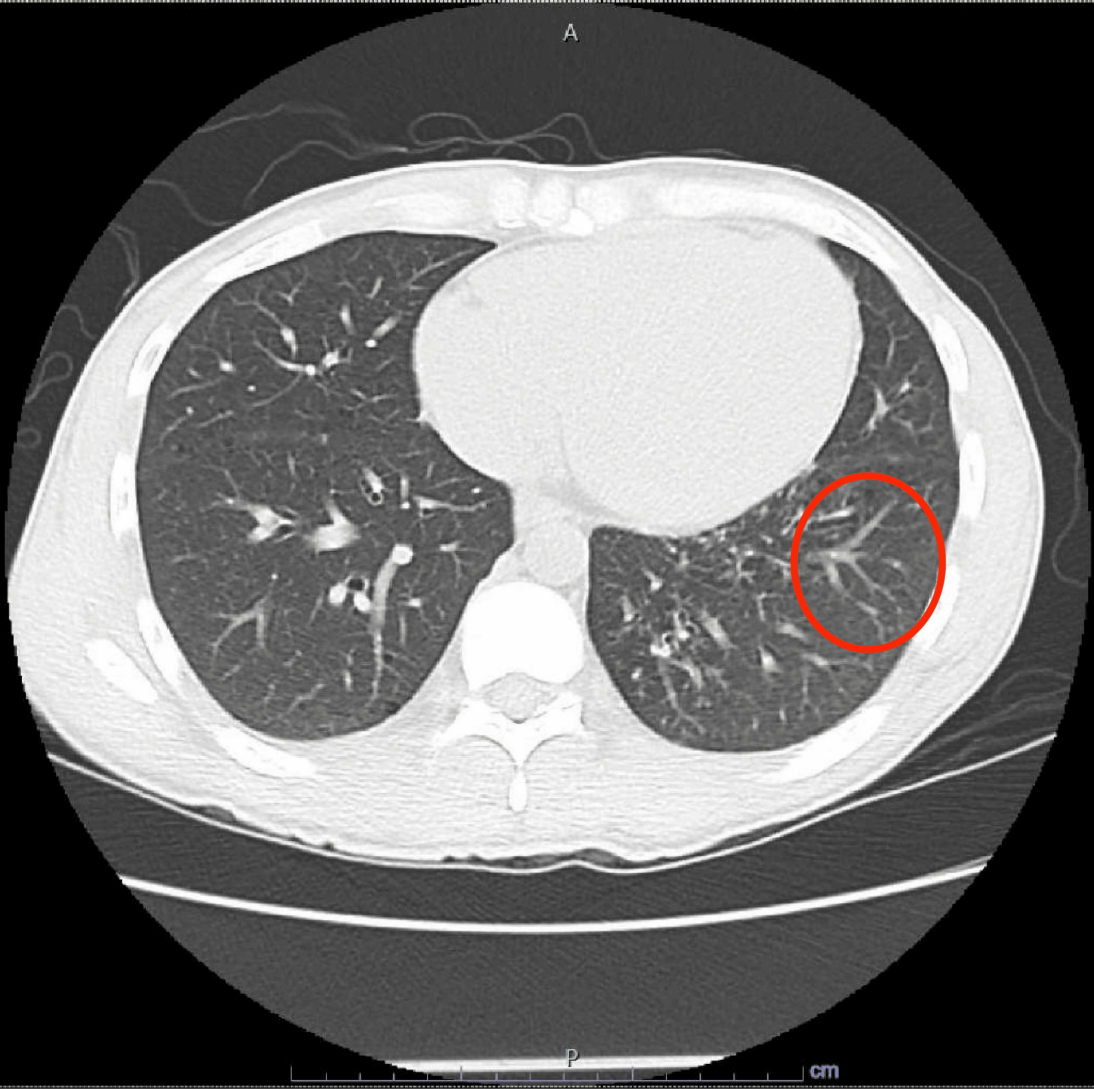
Axial non-contrast chest CT demonstrating a tree-in-bud pattern in the lower lobes. This image depicts the subtle tree-in-bud pattern with centrilobular nodules and branching opacities that give the appearance of budding tree branches, which may be indicative of early pneumonitis. The circled area highlights this finding in the left lung base.
